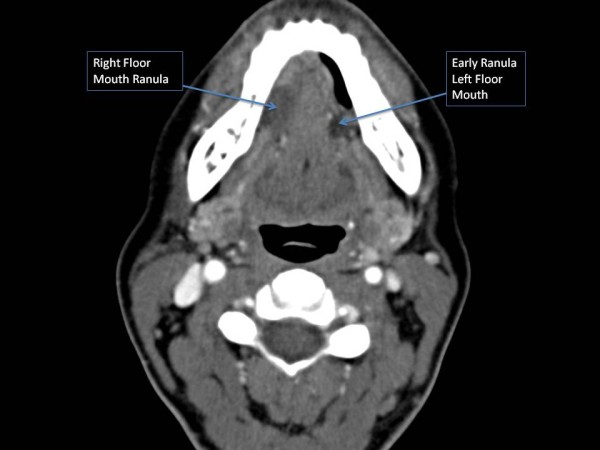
An axial contrast enhanced CT image depicting bilateral floor of mouth ranula.
